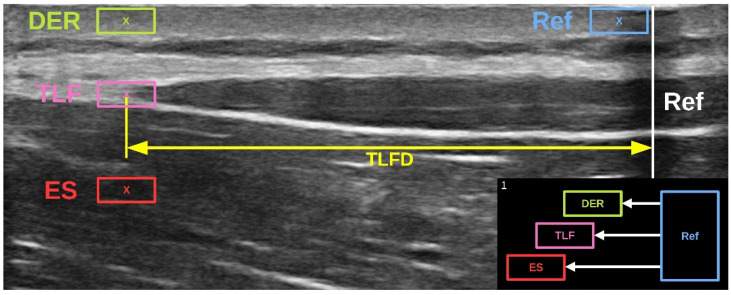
The speckle tracking analysis of lumbar tissue. The respective pixels of the regions of interest (4 × 7 mm rectangles) are tracked in an ultrasound video showing the trunk extension task. The displacement of DER, TLF, and ES from the Ref was post-analyzed using Kinovea software, version 0.9.5. Ref, artificial reference of reflective tape on the skin; DER, dermis; TLF, thoracolumbar fascia; ES, erector spinae muscle; TLFD, deformation of the TLF.
